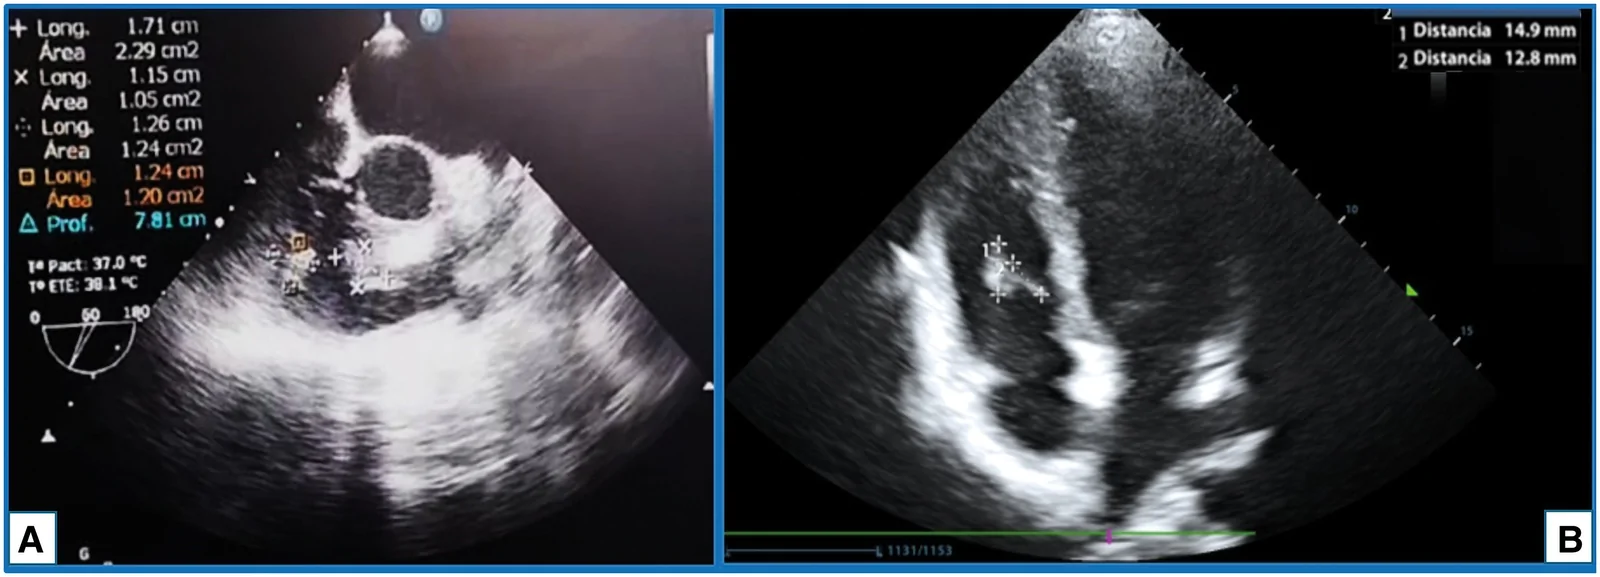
Pre-operative echocardiographic evaluation of the intracardiac metastatic masses. (*A*) Transoesophageal echocardiogram demonstrating multiple heterogeneous, lobulated tumour masses within the right ventricular outflow tract and tracking towards the pulmonary artery trunk. (*B*) Transthoracic echocardiogram apical four-chamber view showing two distinct, mobile, heterogeneous masses firmly attached to the tricuspid valve and subvalvular apparatus.
